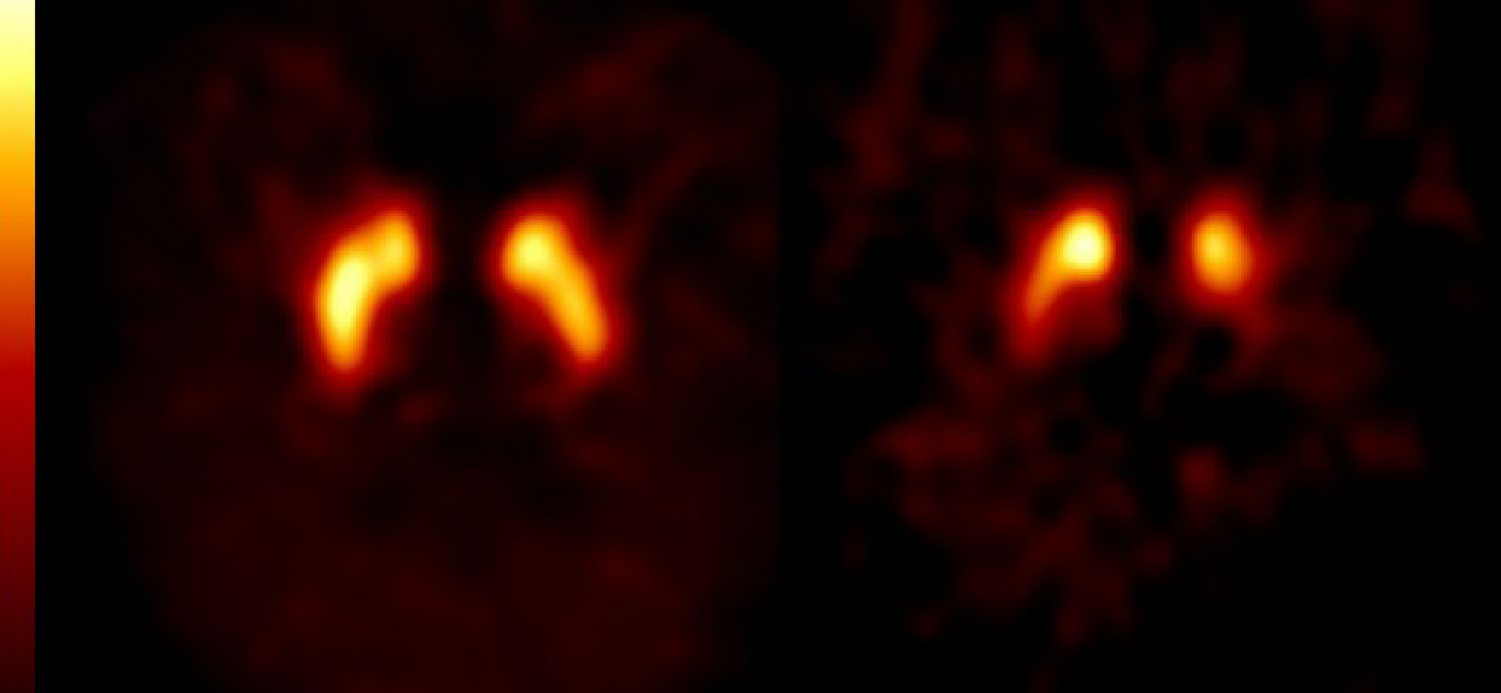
Transversal [^123^I]I-FP-CIT SPECT images at the level of the striatum. Left panel: no loss of striatal binding in a patient without nigrostriatal dopaminergic degeneration. Right panel: loss of striatal binding in a patient with nigrostriatal dopaminergic degeneration. Note the asymmetric striatal binding, and lower binding in the putamen than in the caudate nucleus, which is typical for diseases like Parkinson’s disease. Scans were acquired on a brain-dedicated SPECT system (InSpira) at the Amsterdam UMC. Reprinted from [37].
